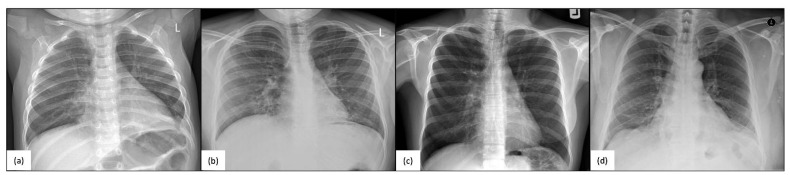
Chest X-rays (CXR) of homozygous patients with PCD for the *RSPH4A* (c.921+3_921+6delAAGT (intronic)) founder mutation. (a) A one-year-old male showing air trapping, (b) a 13-year-old female with perihilar infiltrates, (c) a 38-year-old female with bibasilar atelectasis, and (d) a 51-year-old male with worsening bibasilar atelectasis and fibrotic tissue. No laterality defects are evident. Panel organized using BioRender.com (accessed on 24 November 2022).
